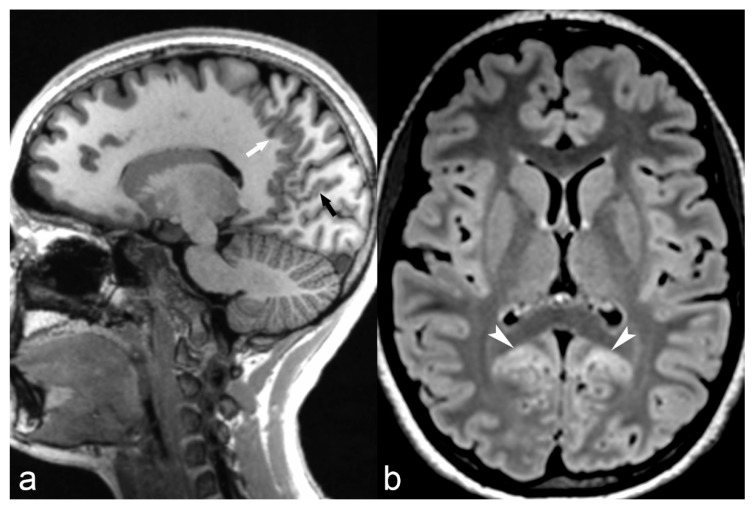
14-year-old female with parieto-occipital polymicrogyria. Sagittal T1 magnetization/prepared-2-rapid-acquisition-gradient-echo (MP2RAGE) (a) image showing numerous small gyri involving the parasagittal parieto-occipital region (arrow) and calcarine sulcus (black arrow) with mild cortical hyperintensity (arrowheads) on axial FLAIR (b) images suggestive of polymicrogyria.
